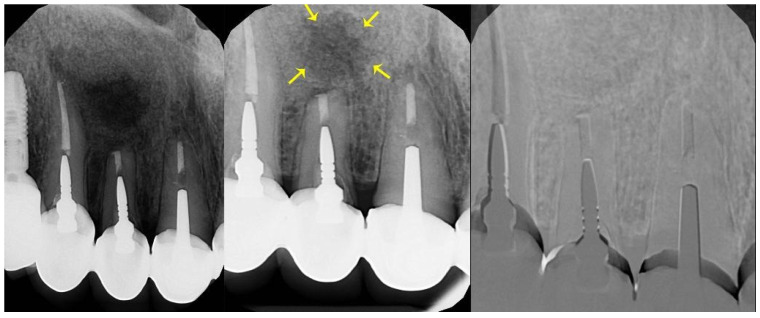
Baseline, follow-up (after 12 weeks), and subtracted radiographs of an upper lateral incisor. Yellow arrows point to newly formed trabeculae oriented towards the centre of the lesion.
